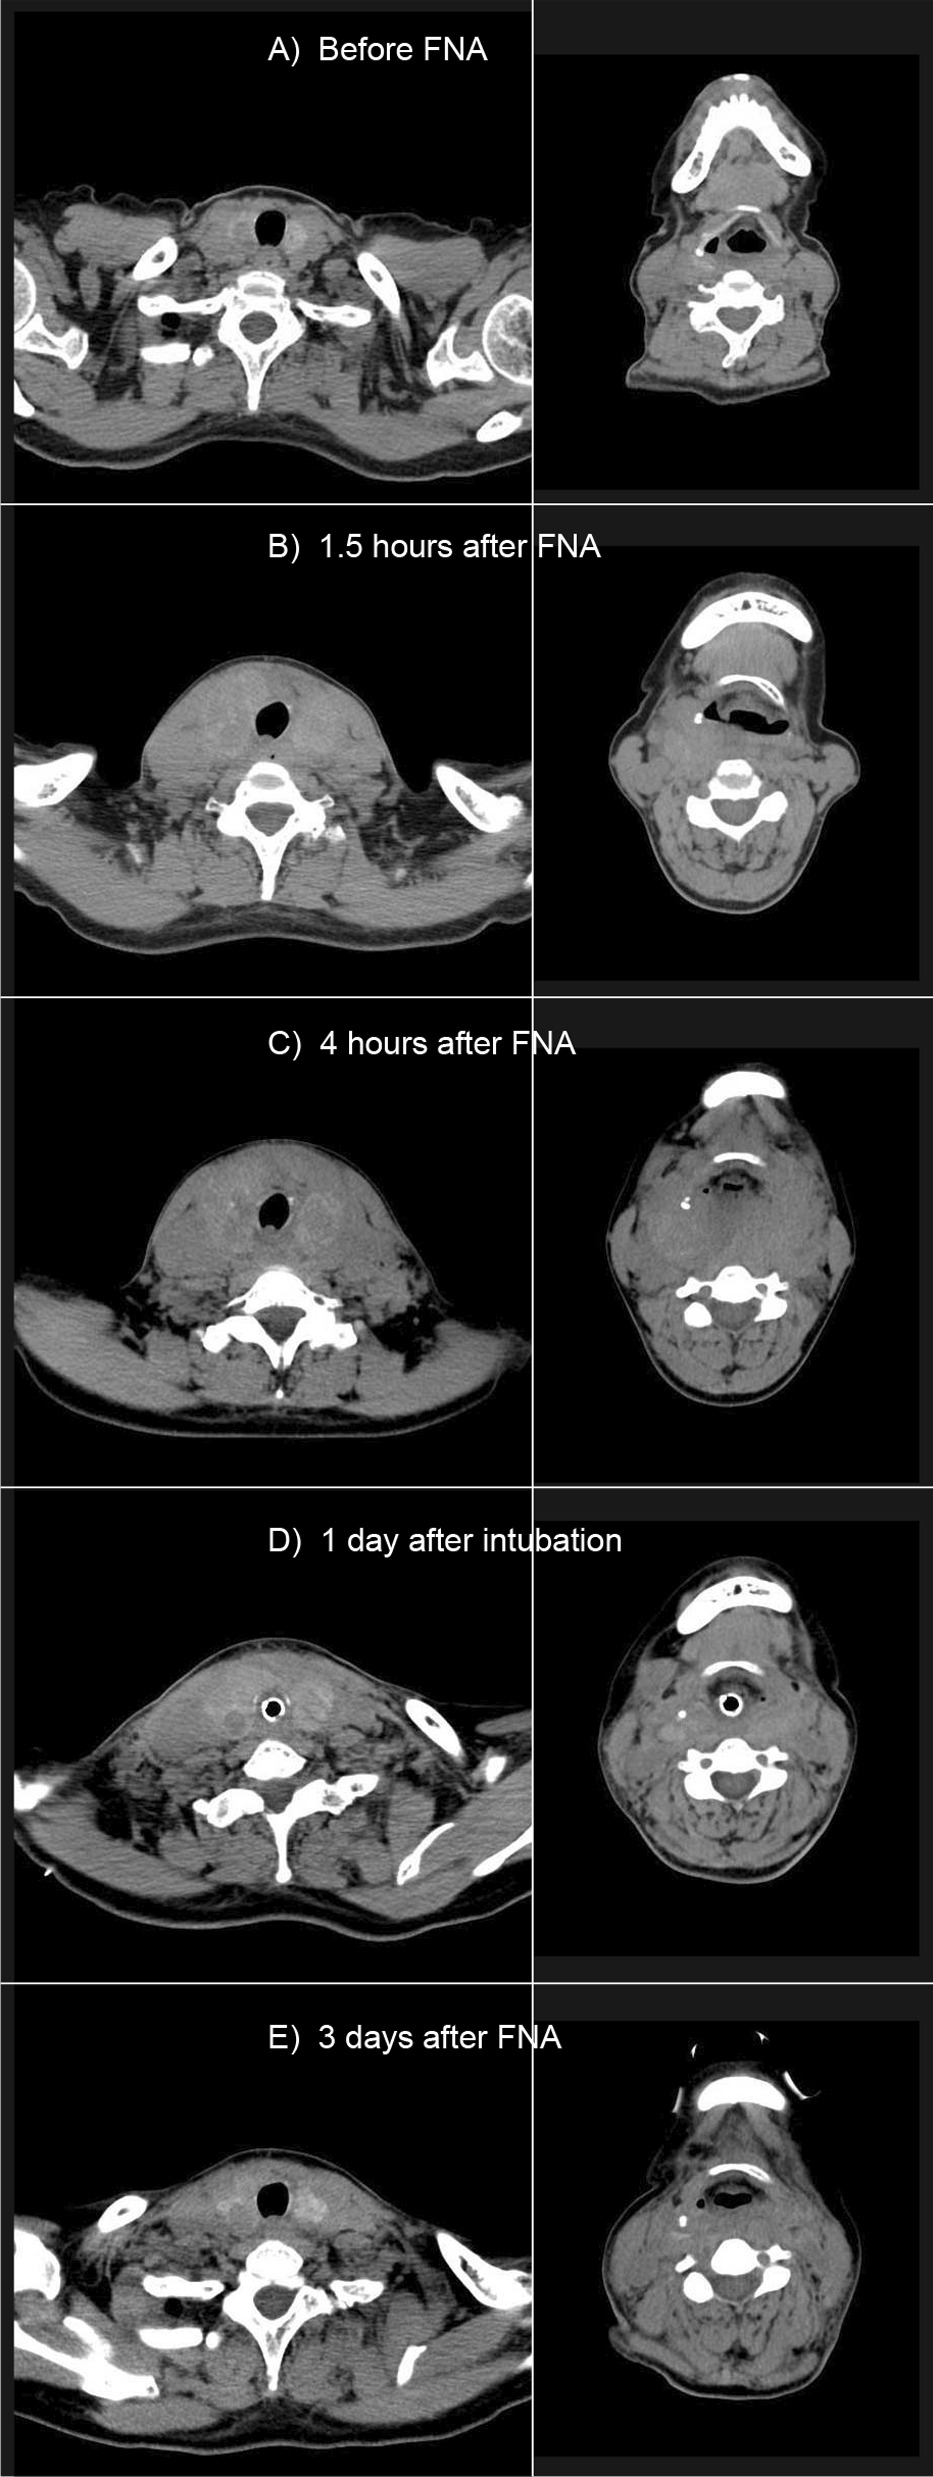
A CT findings before FNA. The thyroid nodule in the right lobe shows low signal intensity compared with surrounding thyroid gland tissue. The right internal jugular vein is dilated. The left panel shows a CT slide obtained at the thyroid gland level, while the right panel shows a slice at the hyoid bone level. B CT findings 1.5 h after FNA. There is swelling not only of the thyroid gland but also of the retropharyngeal, especially on the right side. The right internal jugular vein is dilated. C CT findings before intubation (4 h after FNA). Expansion of the retropharyngeal tissue has increased bilaterally, and the narrowness of the retropharyngeal space is evident. The right internal jugular vein is dilated. D CT findings 1 day after intubation. Swelling of the retropharyngeal tissue has subsided dramatically, and thyroid gland enlargement has decreased. The right internal jugular vein is still dilated. E CT findings 3 days after FNA. Swelling of the retropharyngeal tissue has resolved, and thyroid gland enlargement has further decreased. The right internal jugular vein was continuously dilated
